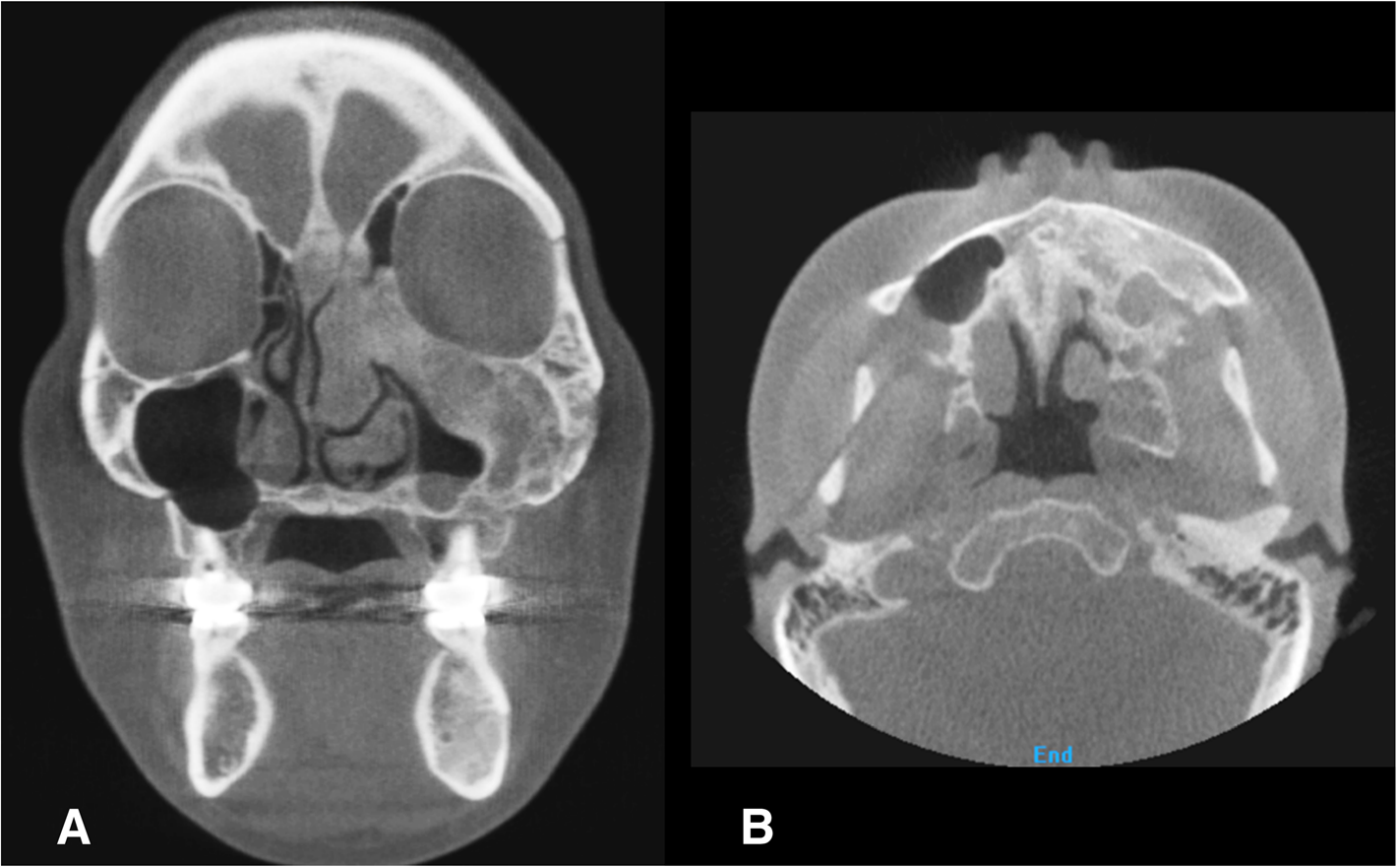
3D CT image of coronal (a) and axial (b) view 4 years postoperative. No further expansion or re-growth of the dysplastic lesion was noted
